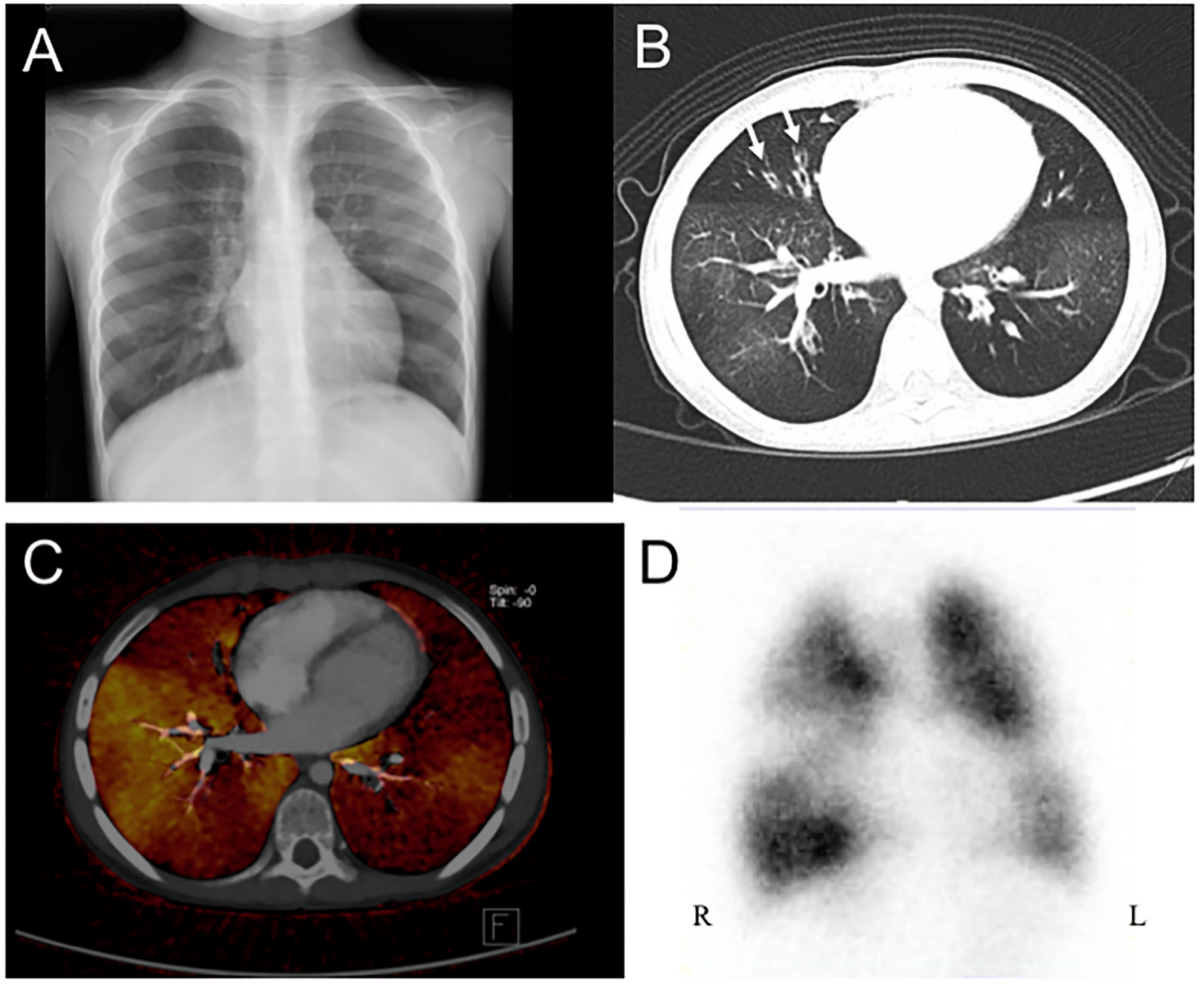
A 4 years old girl diagnosed with post-infectious with initial clinical symptoms of cough and dyspnea. (A) Chest X-ray revealed peribronchial thickening and emphysema. (B) Axial view of HRCT revealed mosaic pattern and bronchiectasis (arrow). (C) Axial view of DECT reveal regional decreased pulmonary blood vasculature, and (D) Perfusion scintigraphy showed reduction blood flow in bilateral lungs. HRCT, high resolution computed tomography; DECT, dual energy computer tomography; R, right side; L, left side.
